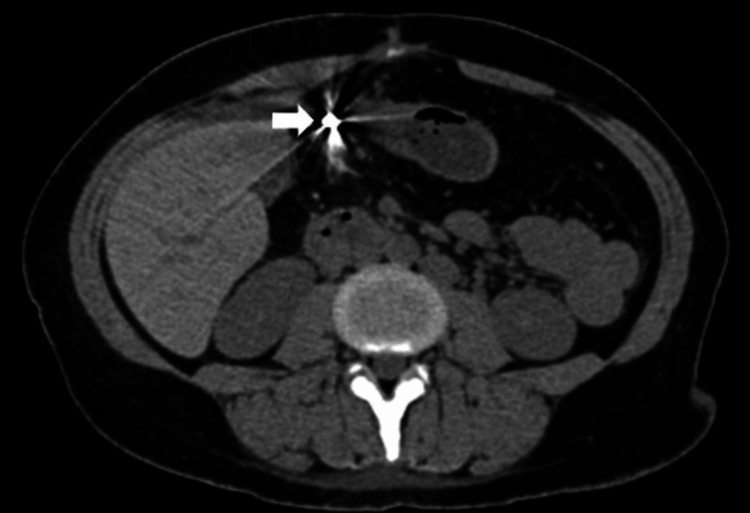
Abdominal CT image demonstrating the apparent coil (white arrow) with almost absence of the collections.
